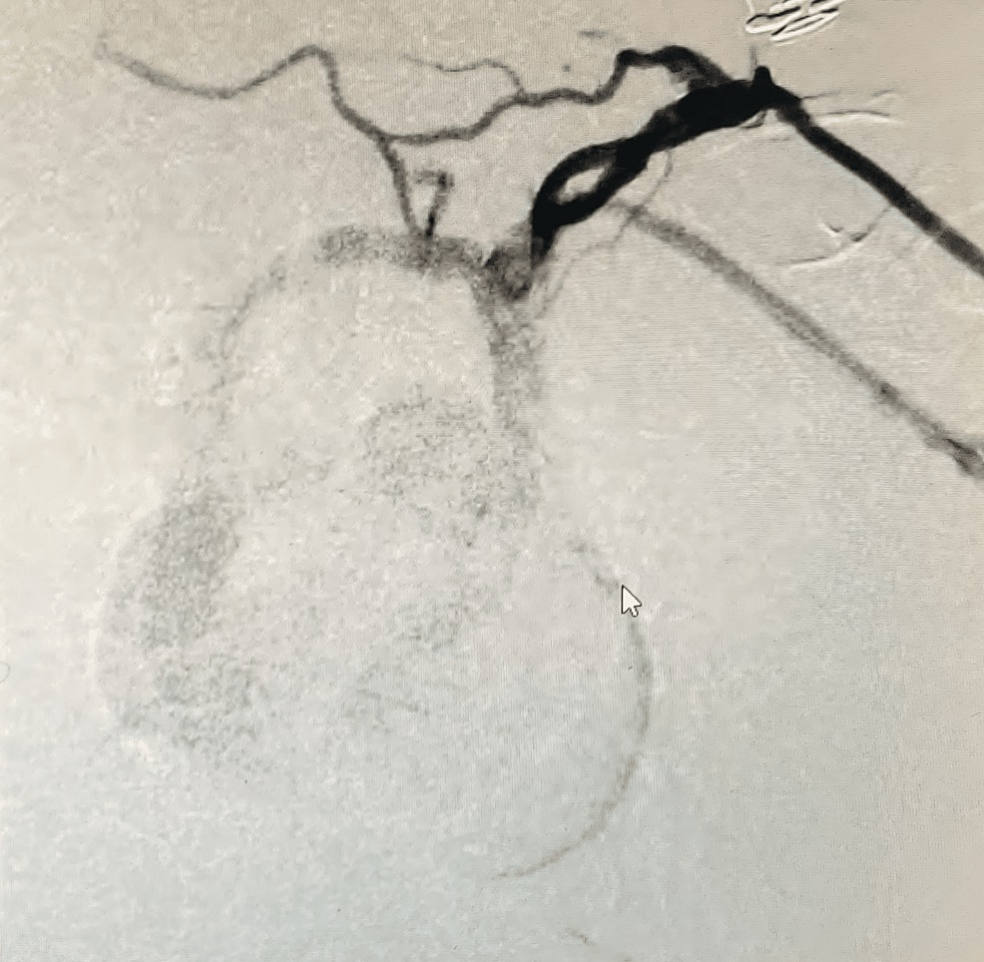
CT Angiography Showing Dilated UCS and PLSVC UCS: Unroofed coronary sinus; PLSVC: Persistent left superior vena cava The image has been taken by the authors.
